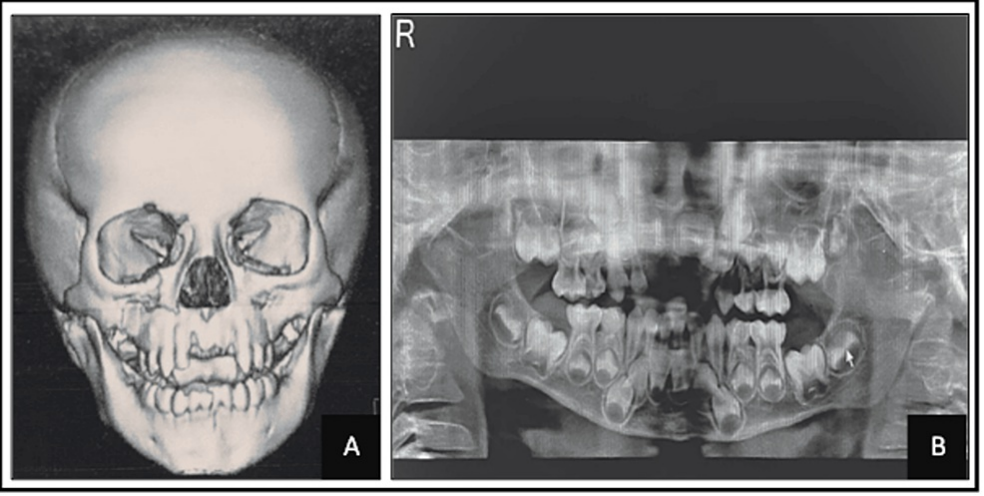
Preoperative intraoral image: (A) CT brain and face; (B) OPG. OPG, orthopantomography; CT, computed tomography
